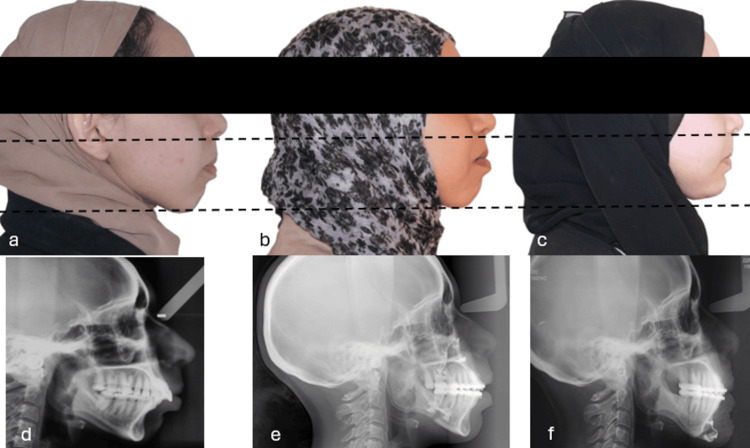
Treatment progression. (a) Pre-treatment profile photograph. (b) Profile photograph after bimaxillary orthognathic surgery. (c) Final profile photograph after secondary genioplasty and completion of treatment. (d) Pre-treatment lateral cephalometric radiograph. (e) Lateral cephalometric radiograph after bimaxillary orthognathic surgery. (f) Final lateral cephalometric radiograph after secondary genioplasty and completion of treatment.
